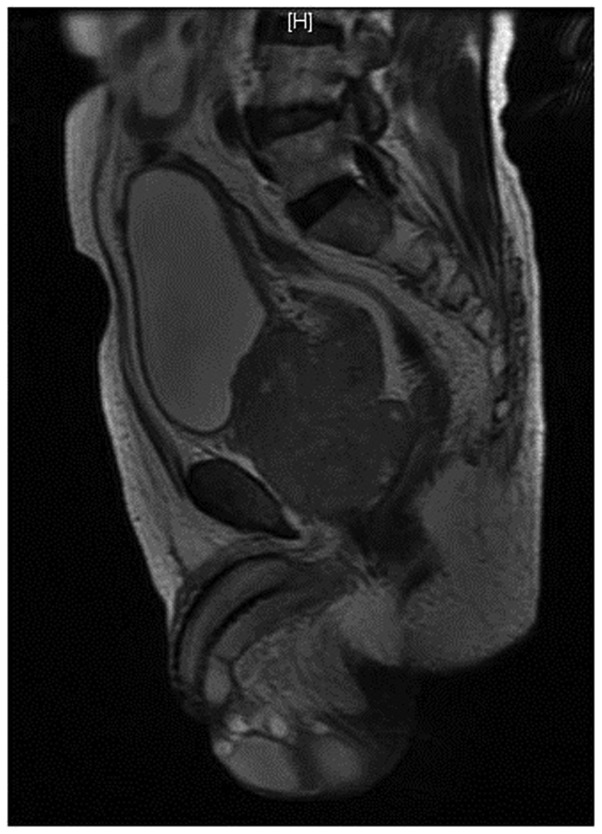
MRI scan showing the prostatic neoplasm, without a neoplasm of the bladder or other areas of the urethra, but with skeletal metastasis.
